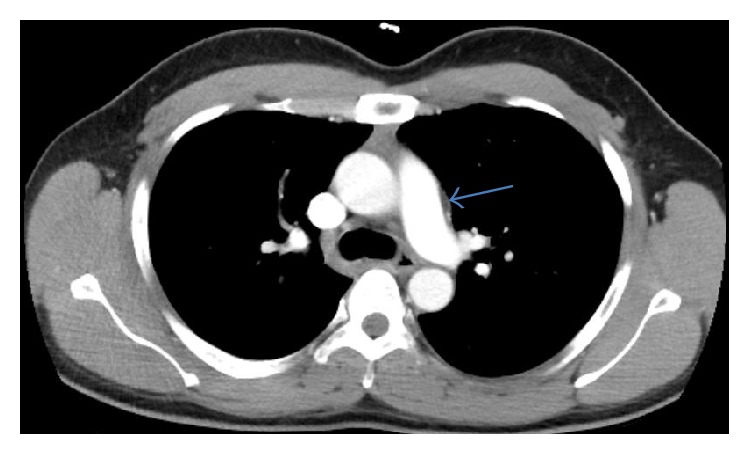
Axial CT scan of the chest (mediastinal window) with regression of mediastinal mass after several months of anti-TB therapy.
